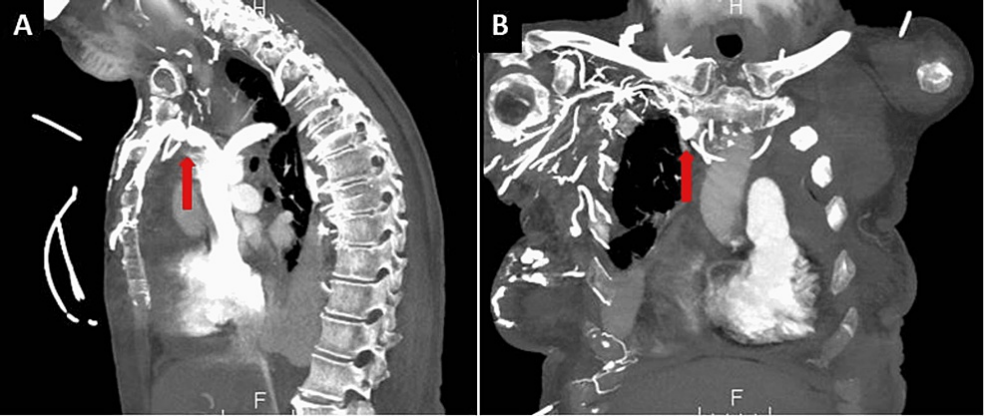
Contrast-enhanced CT scan of the thorax: (A) sagittal view and (B) coronal view demonstrating mediport outside the vascular structures with the tip in the anterior mediastinum, just outside the right brachiocephalic vein (arrows).
